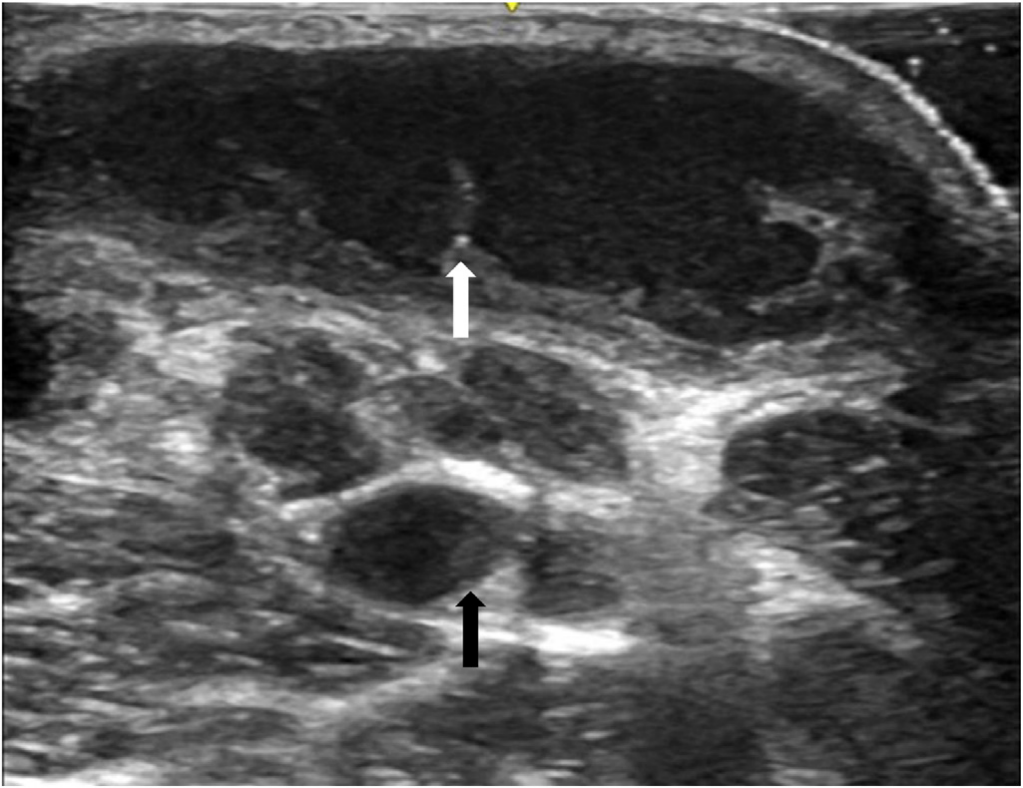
Point-of-care ultrasound revealing large cystic mass with internal septations (white arrow) and cervical adenopathy (black arrow).
